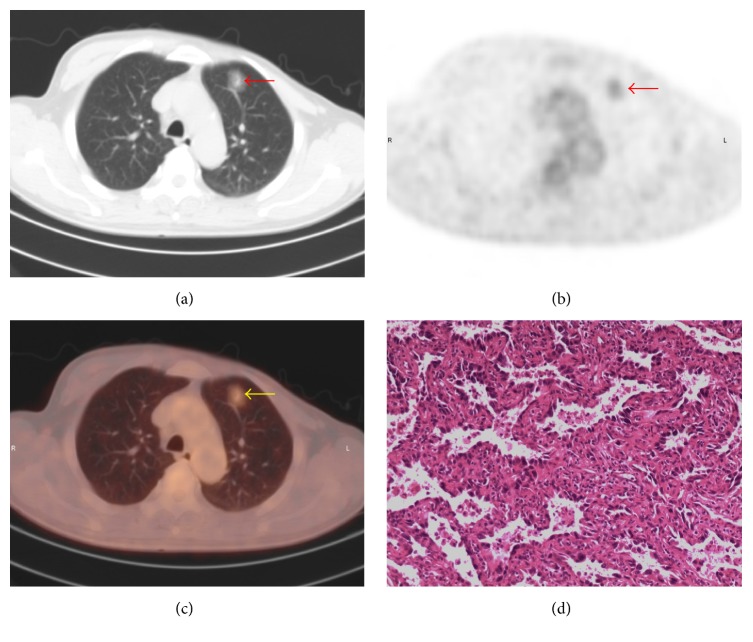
A typical PET/CT finding in a 56-year-old male patient. (a) On CT image, the nodule in the left upper lobe with high density in the center and low density in the surrounding tissue and a clear border is observed (arrow); the maximum diameter of nodule is 22 mm. (b) On PET and (c) on PET/CT image, SUV_max_ = 1.7. (d) Pathologic examination suggests bronchioloalveolar carcinoma. The *y* value calculated by our model is 0.09. The result of our model is inconsistent with PET/CT and pathologic result.
